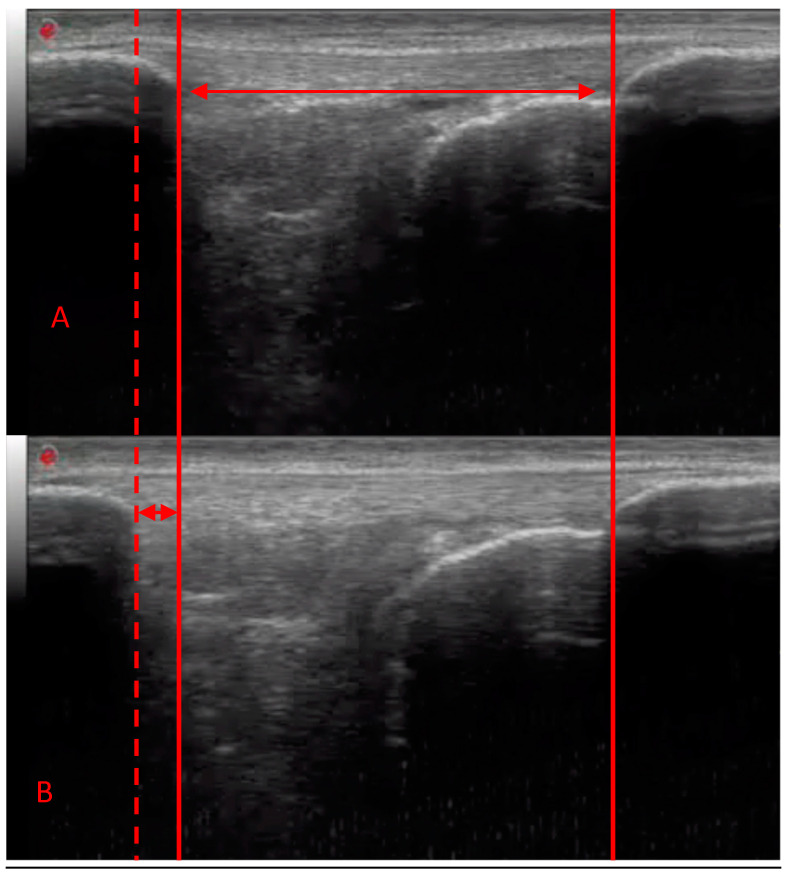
Ultrasonography images of the patellar tendon obtained at rest (A) and during a maximal isometric ramped contraction (B). The solid lines demonstrate the resting tendon length as measured between the apex of the patella and the tibial tuberosity. The dashed line represents the elongation of the tendon that has occurred during contraction.
